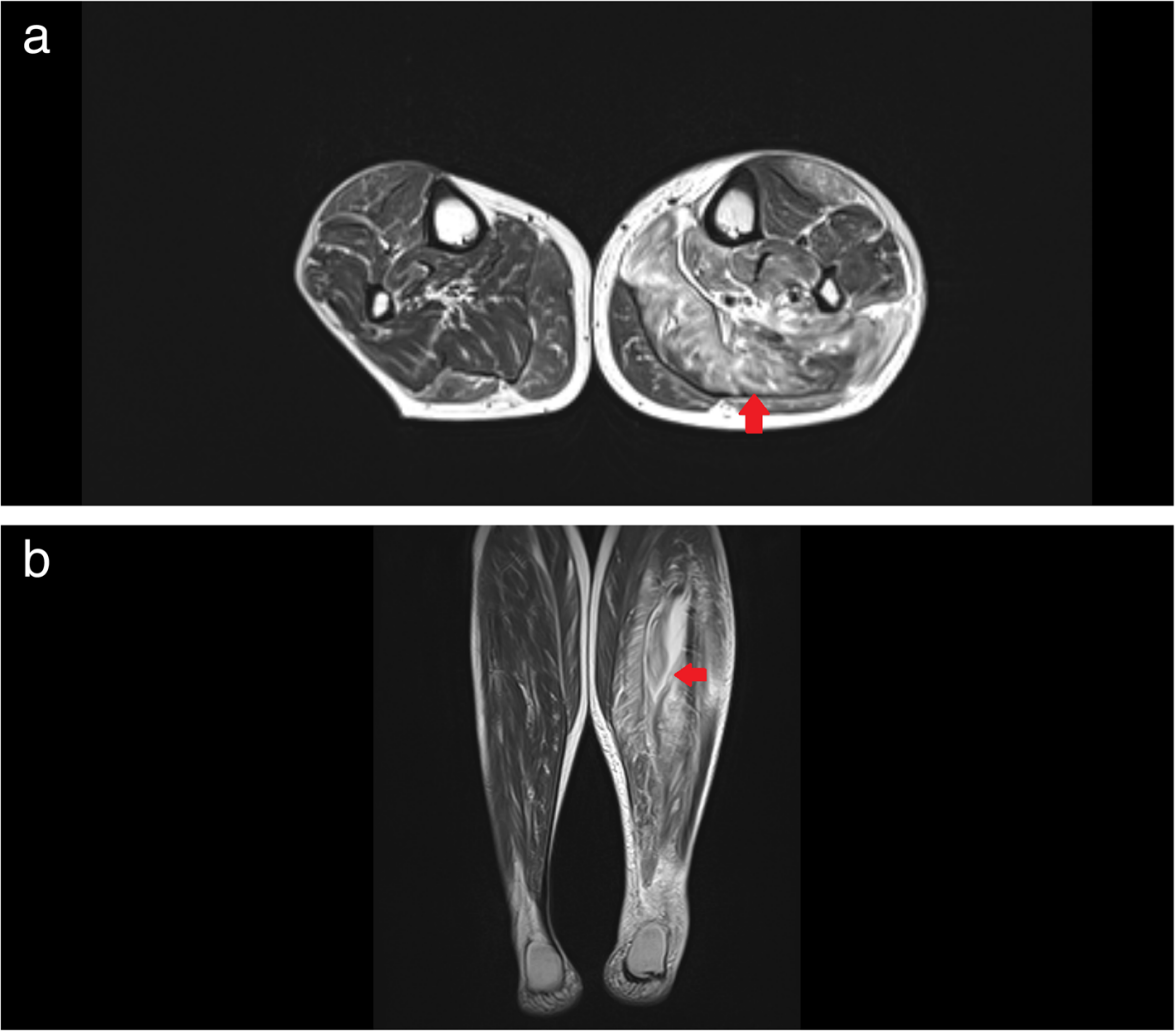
T2-weighted magnetic resonance images of the patient’s lower legs. a. Coronal image; b. Axial image. These images show that the soleus and posterior tibial muscles on the left lower leg (indicated by red arrows) are swollen and inflamed
